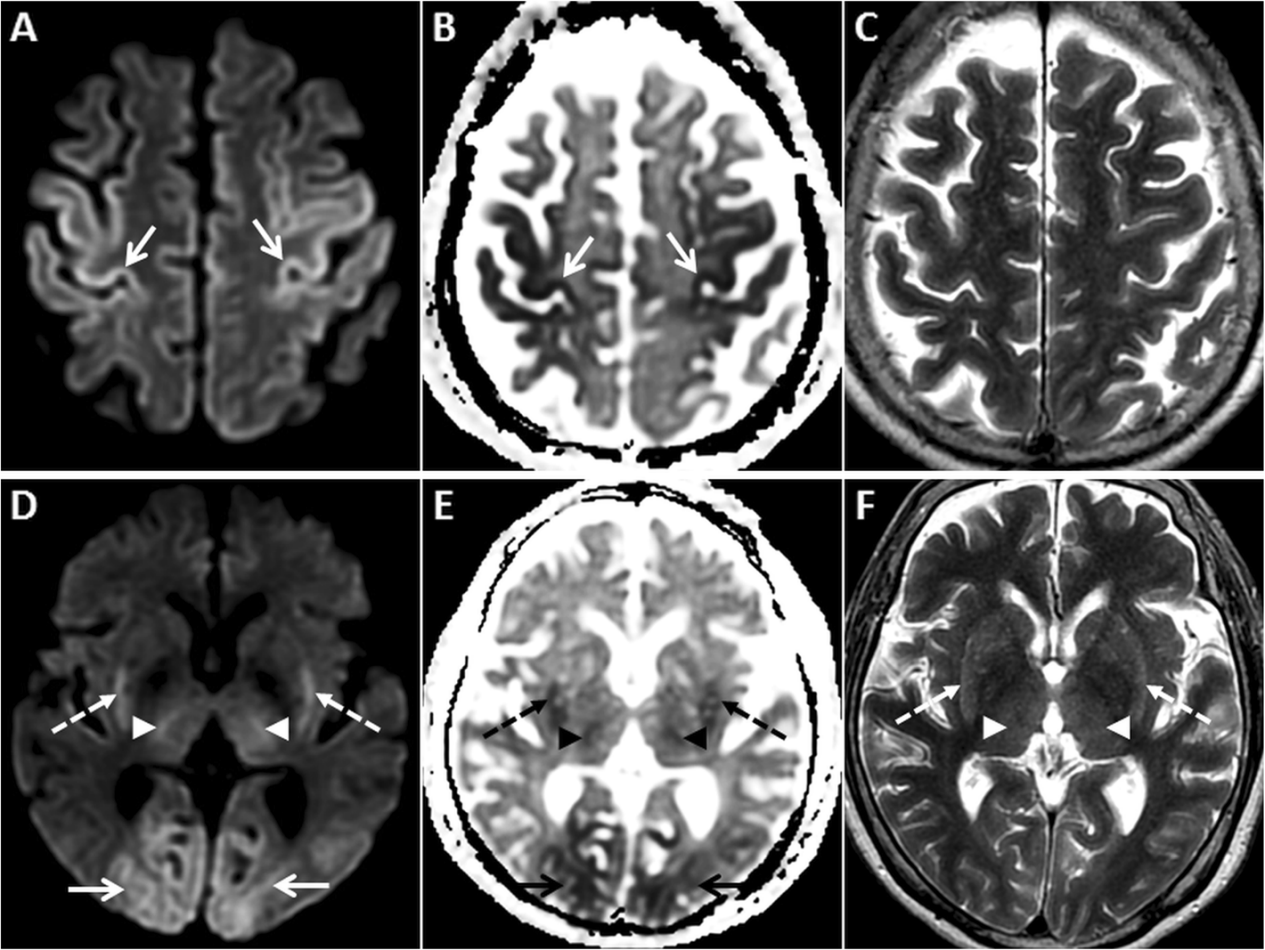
MRI of the brain performed in the acute phase of HIE. On axial DWI (a, d) and axial ADC images (b, e), symmetric GRD is seen in the perirolandic cortices (arrows in a and b) and in the visual cortices bilaterally (arrows in d and e). Restricted diffusion is also observed in the putamina (dashed arrows in d and e) and in the thalami (arrowheads in d and e). Corresponding axial T2W images (c, f) demonstrate no obvious signal abnormality in the cortices. However, there is mild T2 hyperintensity in the putamina (dashed arrows) and in the thalami (arrowheads) (f)
